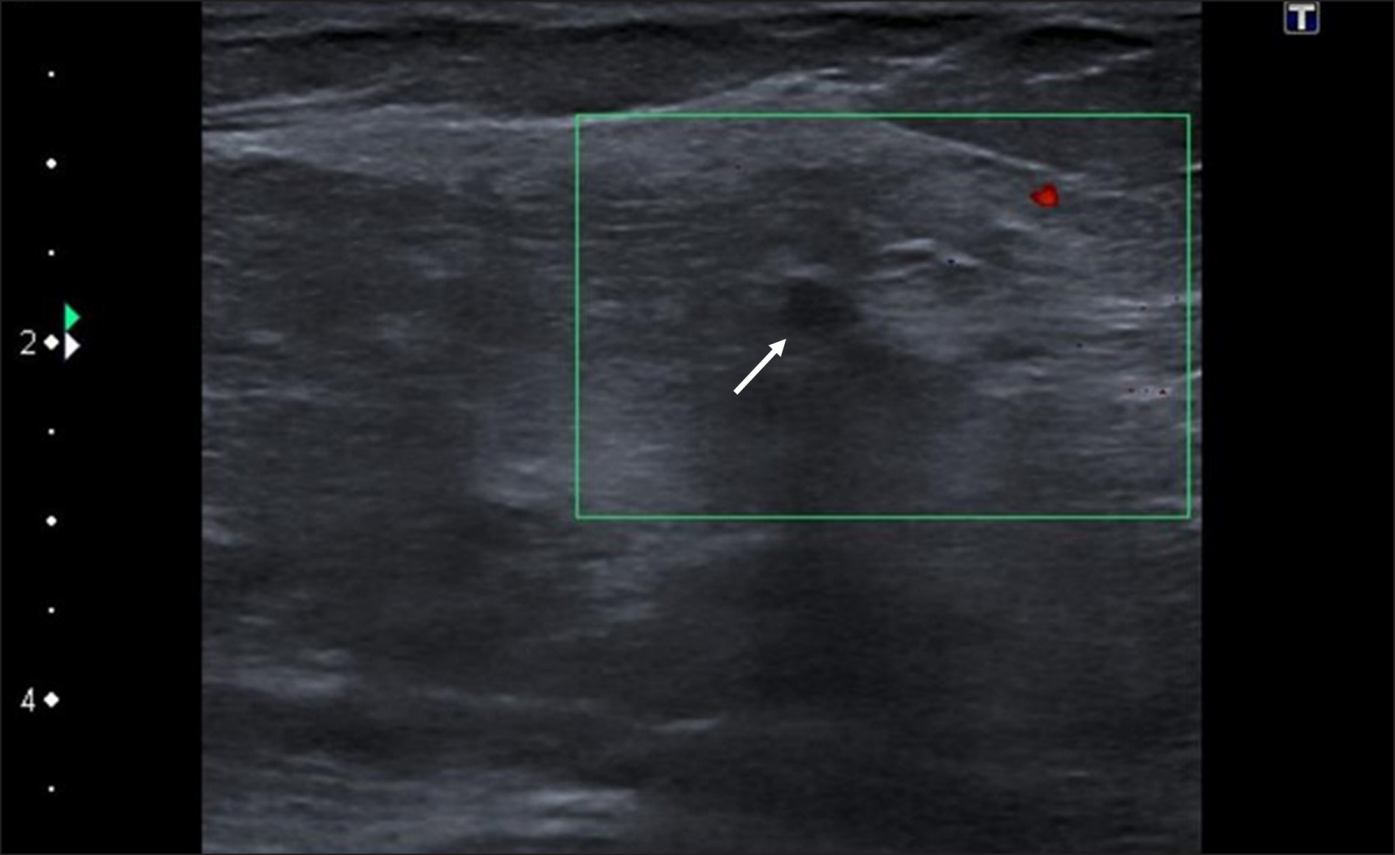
Color Doppler ultrasound. Breast ultrasound of the non-palpable was lesion demonstrated a solid, hypo-echoic mass (arrow) with partially obscured borders. No internal vascularization is identified.
